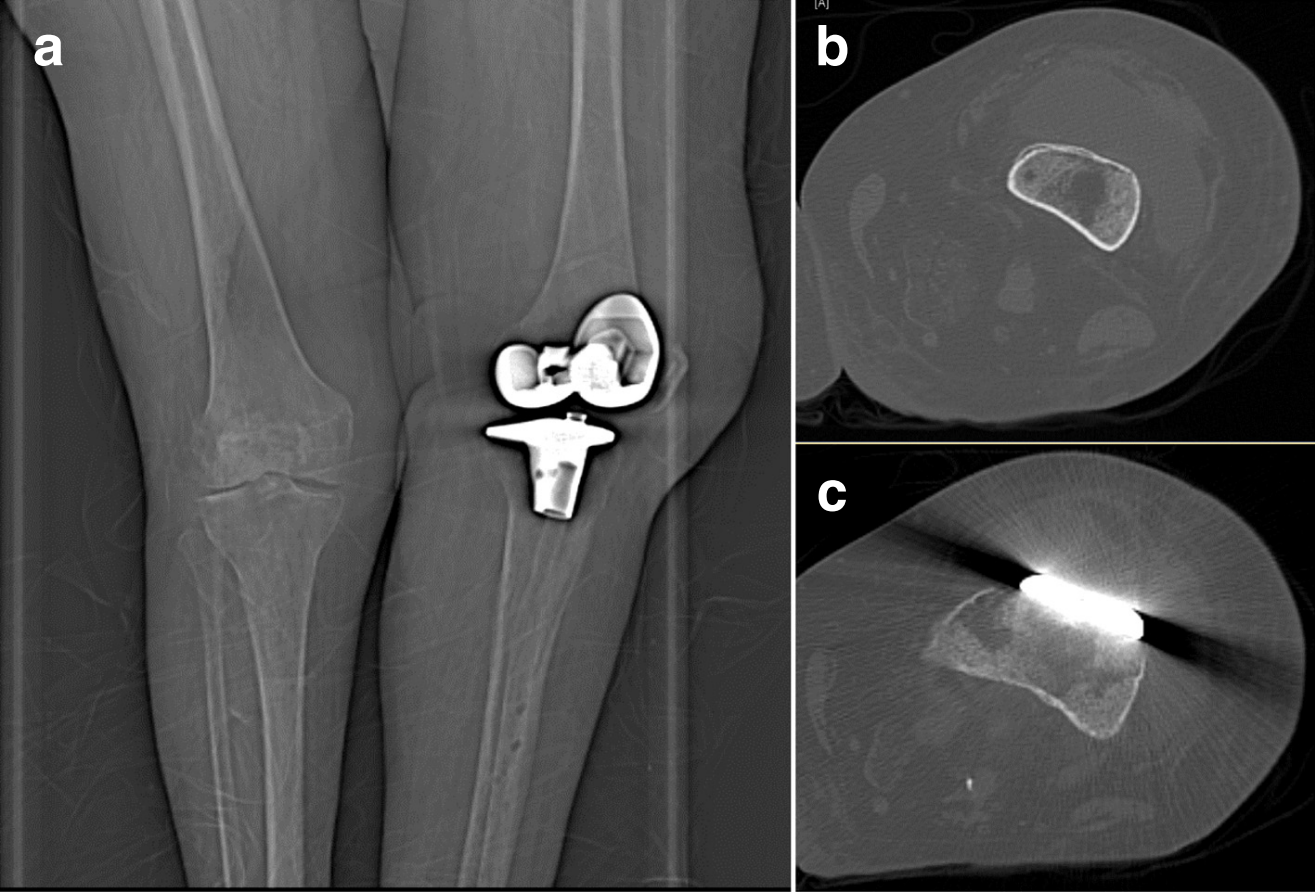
Frontal radiograph of the left knee at admission demonstrates normal post-operative appearance of prosthesis with no prosthetic loosening (a); Computer tomography (CT) shows an abscess in front of the knee prosthesis caused by *Pasteurella multocida* (b and c)
